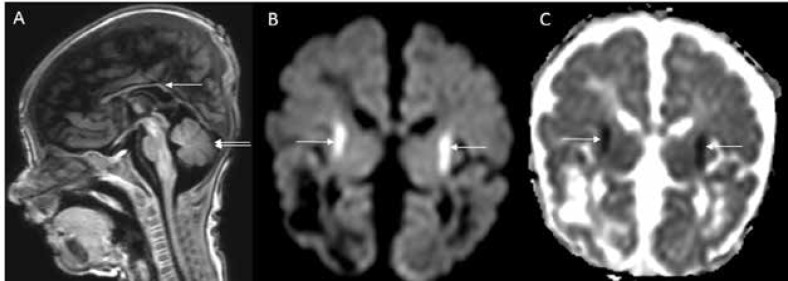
MRI brain T1 sagittal image (A) shows diffuse thinning of corpus callosum (white arrow); brainstem appear normal and cerebellum also appear normal (double arrows). DWI (B) and ADC (C) images show restricted diffusion along corticospinal tracts at the corona radiata (white arrows
